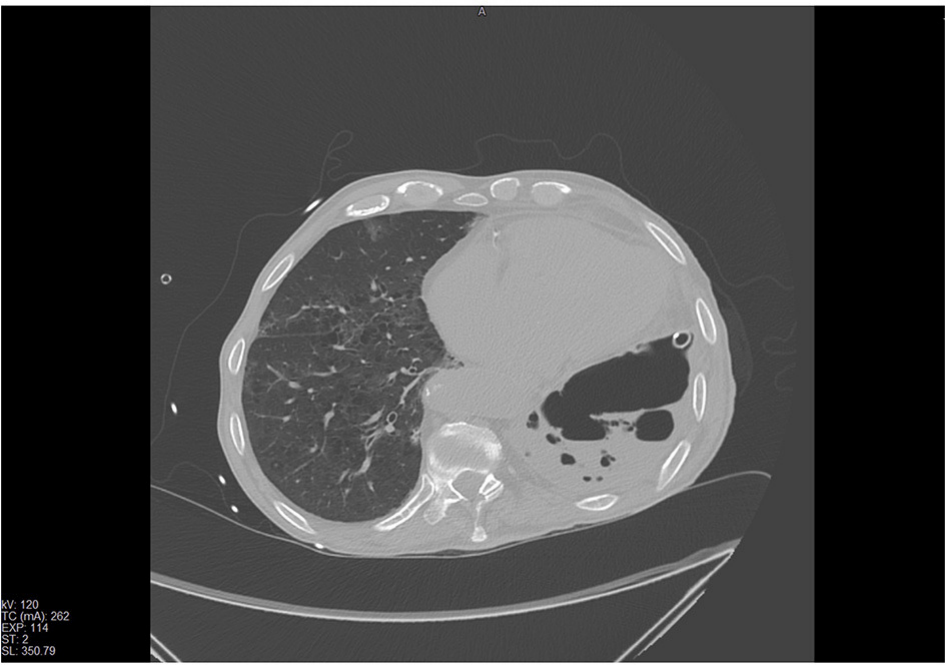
Chest CT scan revealing a non-homogeneous increase in density, diffuse GGO and consolidations at the right lung.
